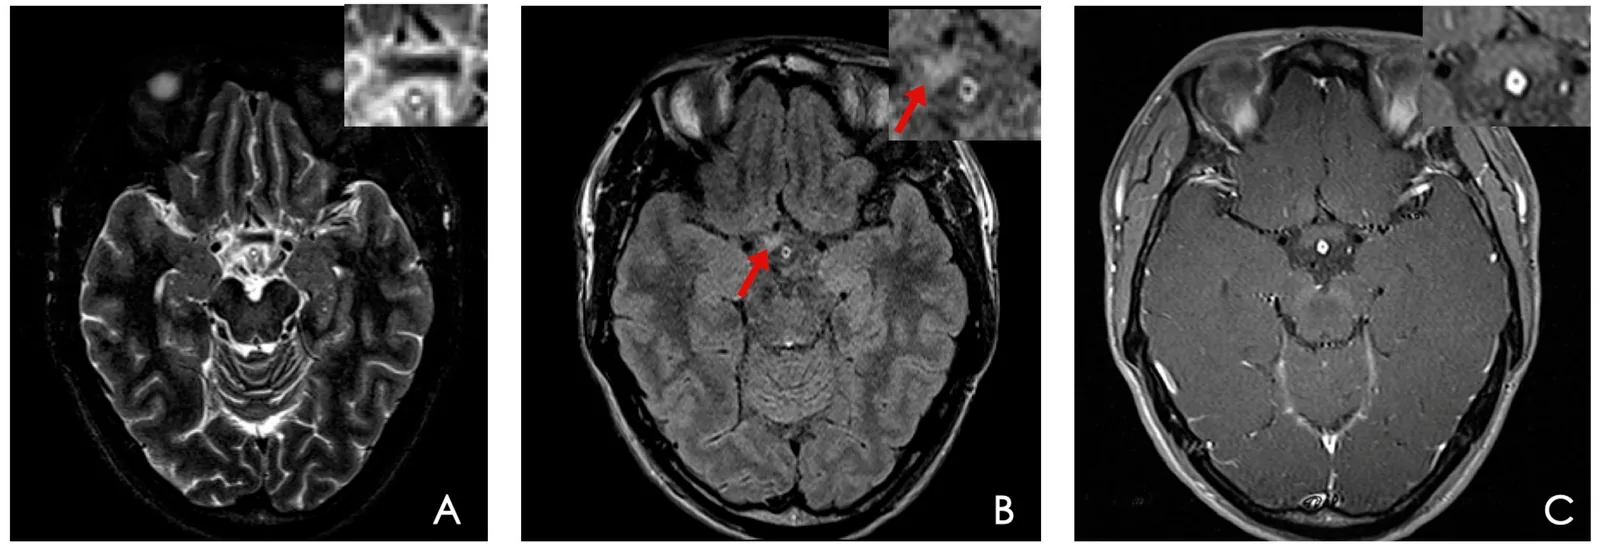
Seronegative ON with a lesion located at the optic chiasma. Axial T2WI-STIR (A) does not show a significant lesion; Axial FLAIR-FS (B) shows a localized high signal at the optic chiasma (red arrow); Axial CE-T1WI (C) shows no corresponding enhancement at the optic chiasma. All panels were displayed at identical magnification and windowing settings to facilitate direct comparison.
